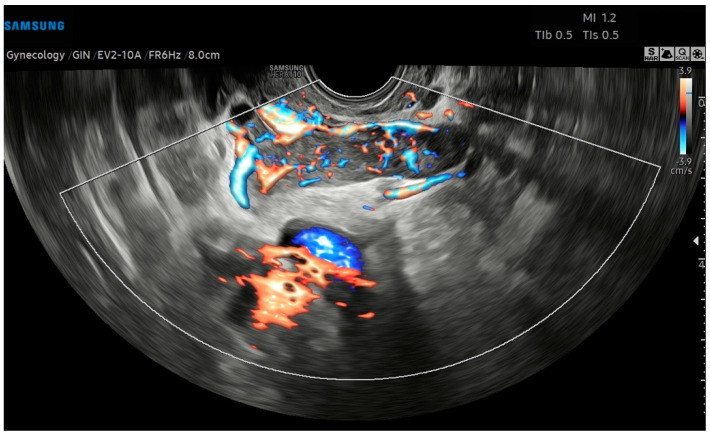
Transvaginal ultrasound with Color Doppler analysis; the longitudinal plane shows a solid elongated, hypoechoic, left parauterine mass. The Color Doppler analysis emphasizes the intense vascularization of the lesion.
